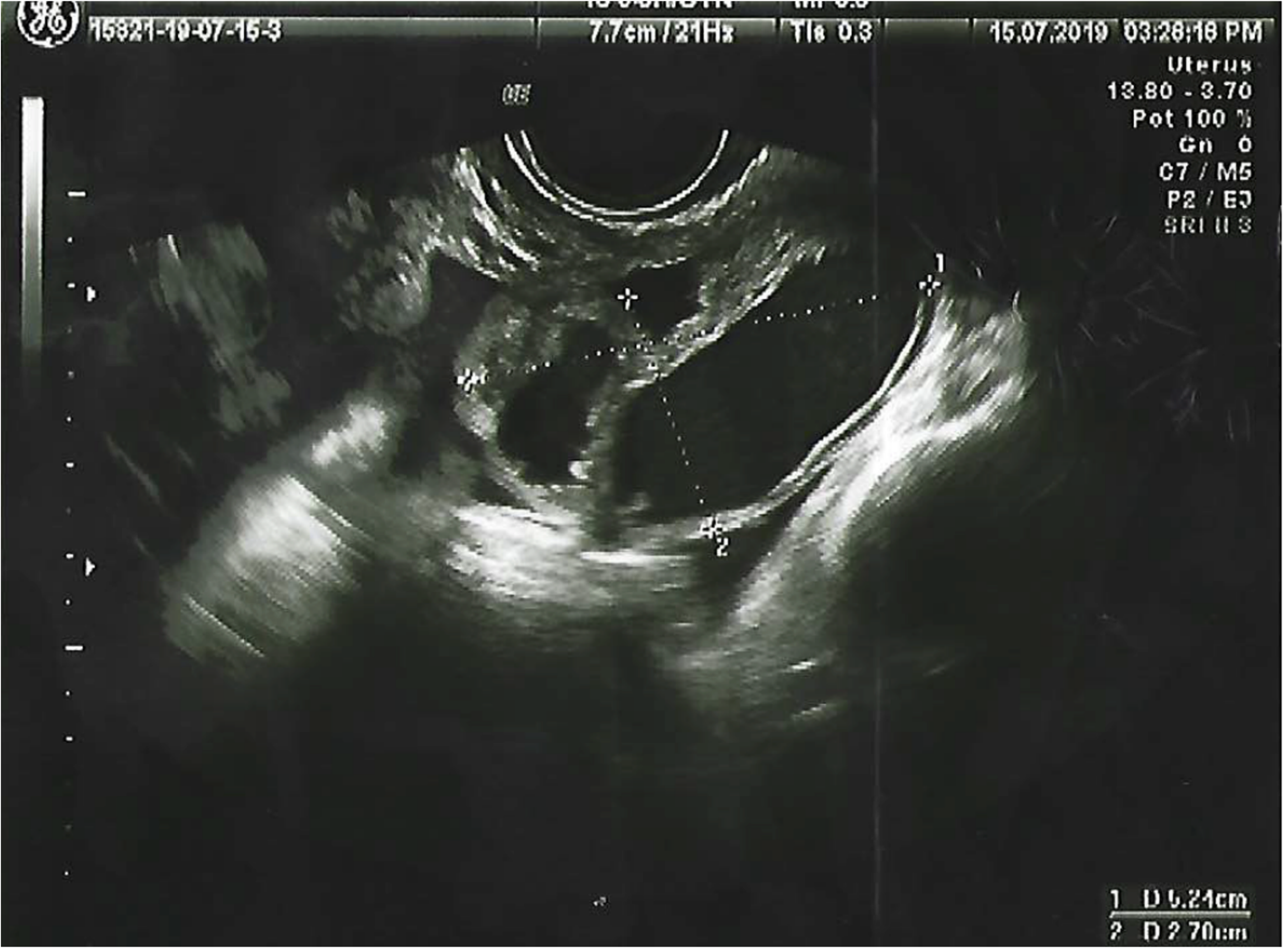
Pelvic ultrasound showing hydrosalpinx
